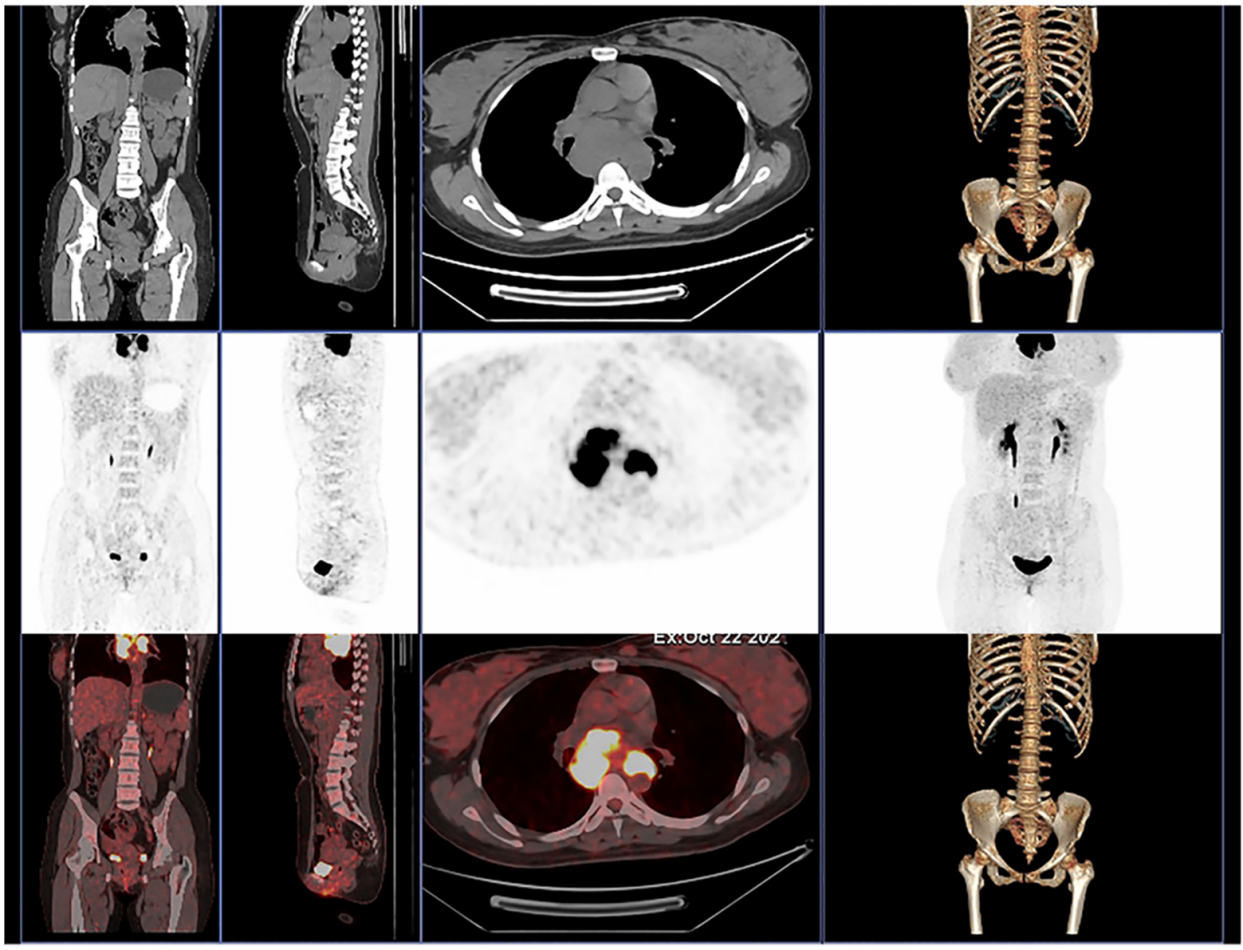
The ^18^F-FDG PET/CT of our patient shows multiple enlarged confluent lymph nodes in the mediastinum with significantly increased glucose metabolism.
